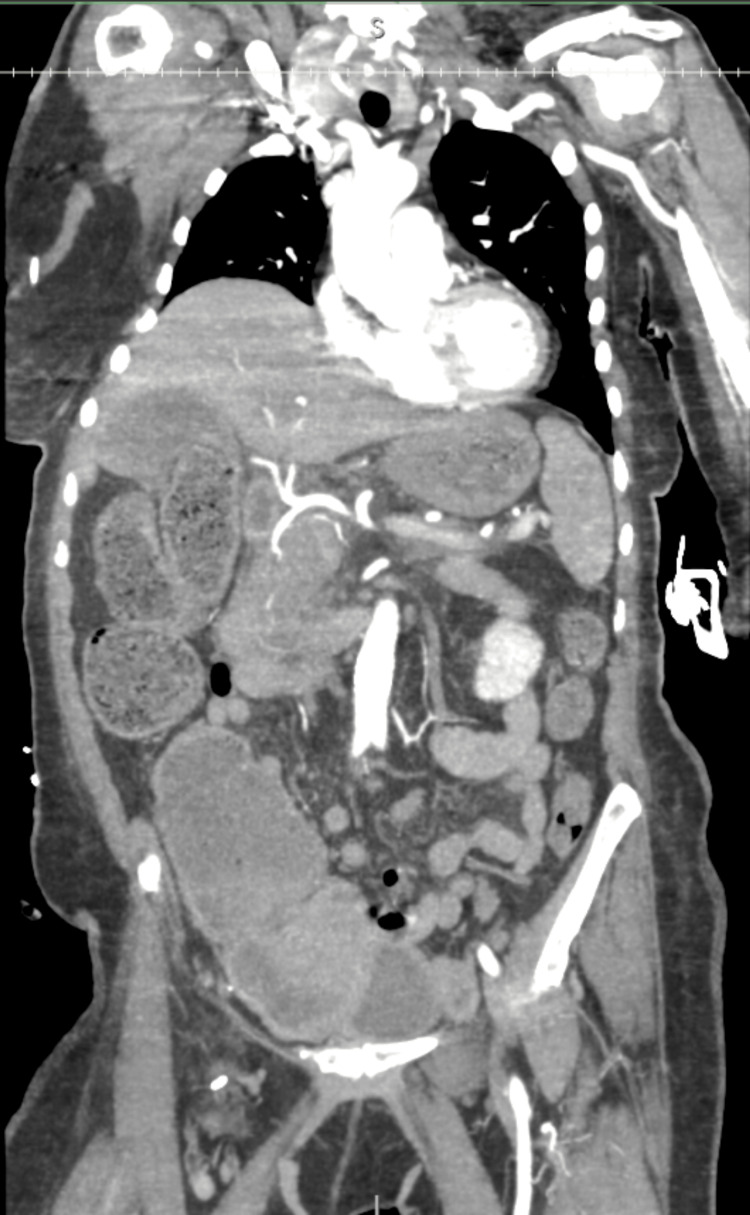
CT Angiogram of the Thorax/Abdomen/Pelvis (Coronal View) CT angiography of the thorax/abdomen/pelvis does not reveal any overt extravasation and is grossly normal. CT: computed tomography
